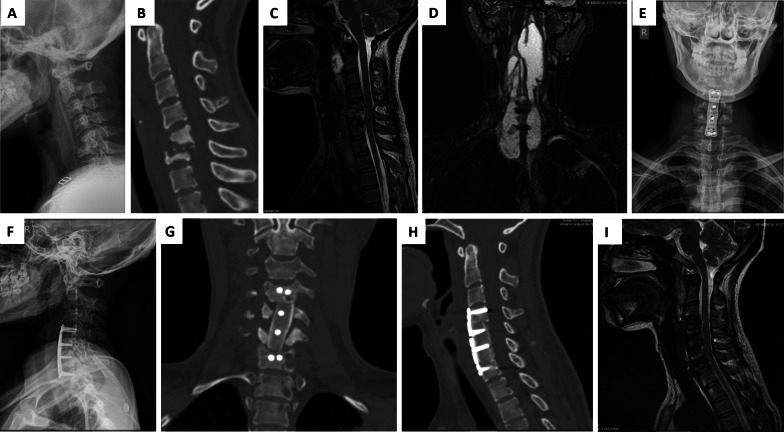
A 39-year-old female patient with cervical tuberculosis. A Preoperative X-ray showing bone destruction in the lower cervical spine and narrowing of the intervertebral space. B Preoperative computed tomography (CT) showing bone destruction, narrowing of the intervertebral space, marked swelling of the prevertebral soft tissue, and formation of dead bone and abscesses. C, D Magnetic resonance imaging (MRI) showing vertebral bone destruction and intraspinal mass leading to significant compression and thinning of the cervical spinal cord. E–I Postoperative X-ray, CT, and MRI showing no loosening or fracture of screws, the implanted iliac crest has fused, the physical curvature of the cervical spine has been restored, and cervical cord compression has been released
